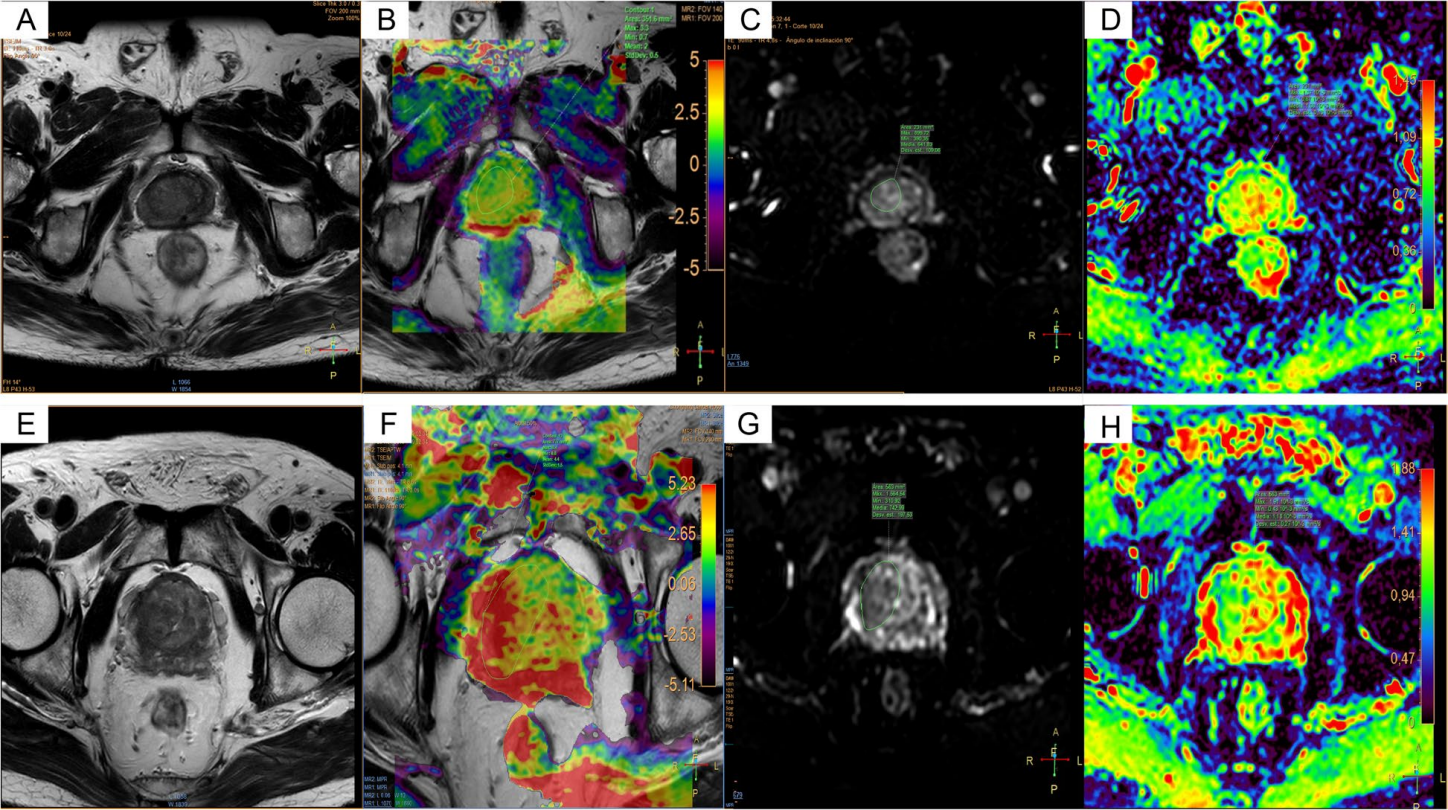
Case examples. 1 A 75-year-old man was hospitalized, and the physical examination revealed that PSA level increased. (1A) T2WI. (1B) APT. (1C) DWI. (1D) ADC. 2 A 68-year-old man was hospitalized for treatment after finding the possibility of prostate malignancy in the external hospital. (2A) T2WI. (2B) APT. (2C) DWI. (2D) ADC
